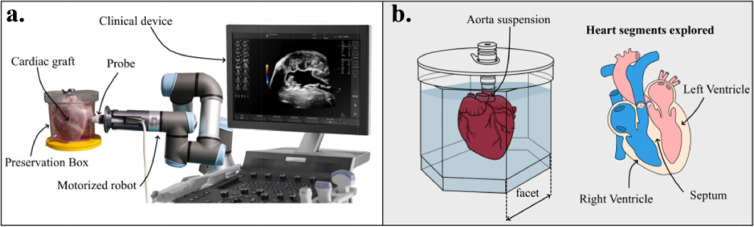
Experimental set-up. a. The heart graft was immerged on an ultrasound transparent box suspended by the aorta. The robot system was used to hold the imaging probe and enable scanning. Three heart structures were explored, the right ventricle, the septum and the left ventricle. b. Preservation box and a slice view of the heart with the target structures for SWE analysis.
